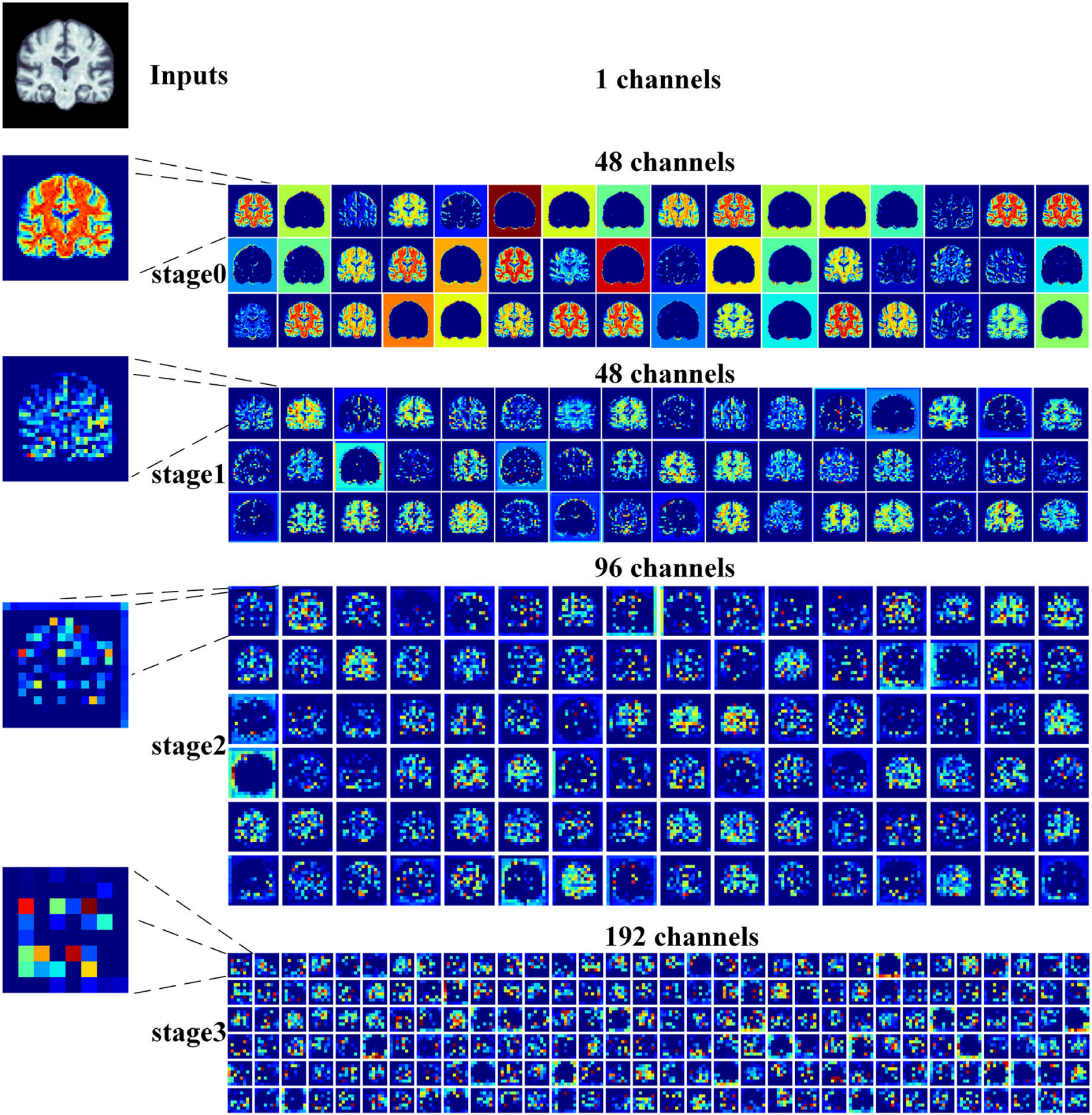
Grad-CAM visualizations of the feature maps produced at different stages by the RepResANet encoder parts. All channel features are derived from one slice of the 3D MRI.
